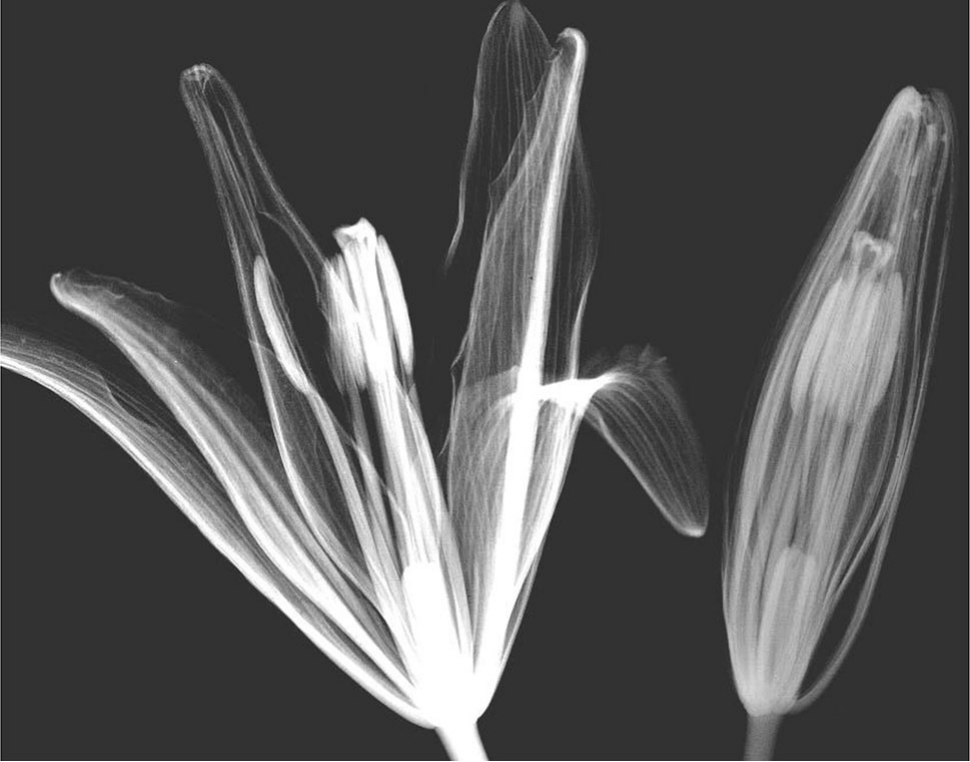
Water image of lily flower and bud by neutron beam. *Note* The degree of penetration of the neutron beam is highly dependent on the amount of water in the sample. The *whiter* part is the place where water content is higher; so the neutron beam could not penetrate the sample well; therefore, the exposure of the X-ray film behind the plants was low, which resulted in whiter image. Through calculation, the amount of water in tissue can be obtained
